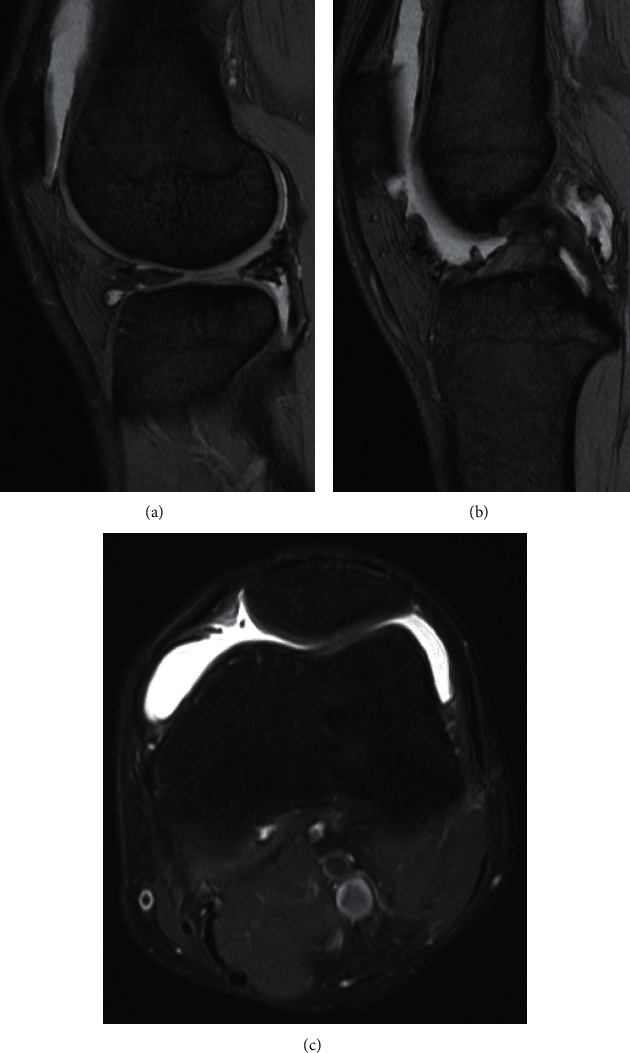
Magnetic Resonance Imaging (MRI) findings at 6 months after the initial surgery (T2-weighed sagittal images). (a) Repaired meniscus appears to be healed. (b) Synovial proliferation is identified around the posterior cruciate ligament and in the posterior compartment. (c) Intra-articular fluid retention is present.
